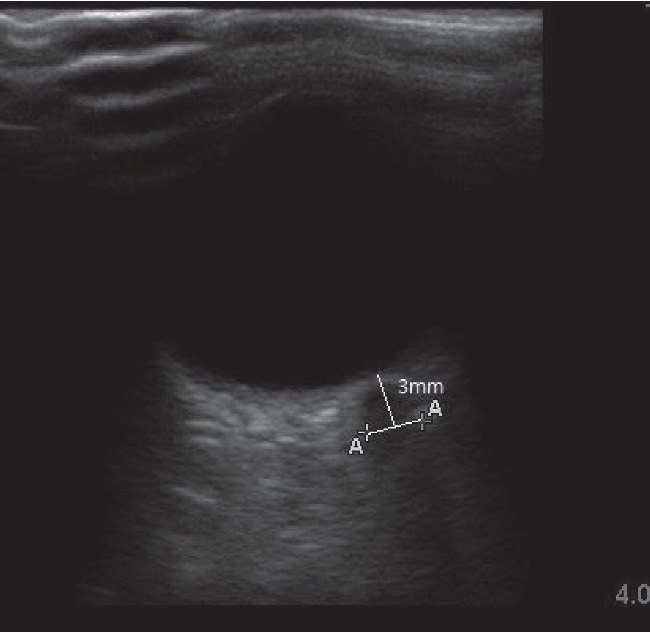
Optic nerve sheath sonography.
